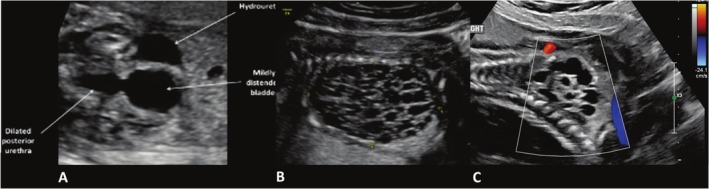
Prenatal ultrasound findings in Case 4. (A) Mildly distended fetal bladder and dilated posterior urethra (“keyhole sign”) at 20 weeks' gestation. (B) Bilateral hyperechogenic kidneys with multiple cortical cysts consistent with renal dysplasia. (C) Color Doppler confirming severe parenchymal damage with absent corticomedullary differentiation.
